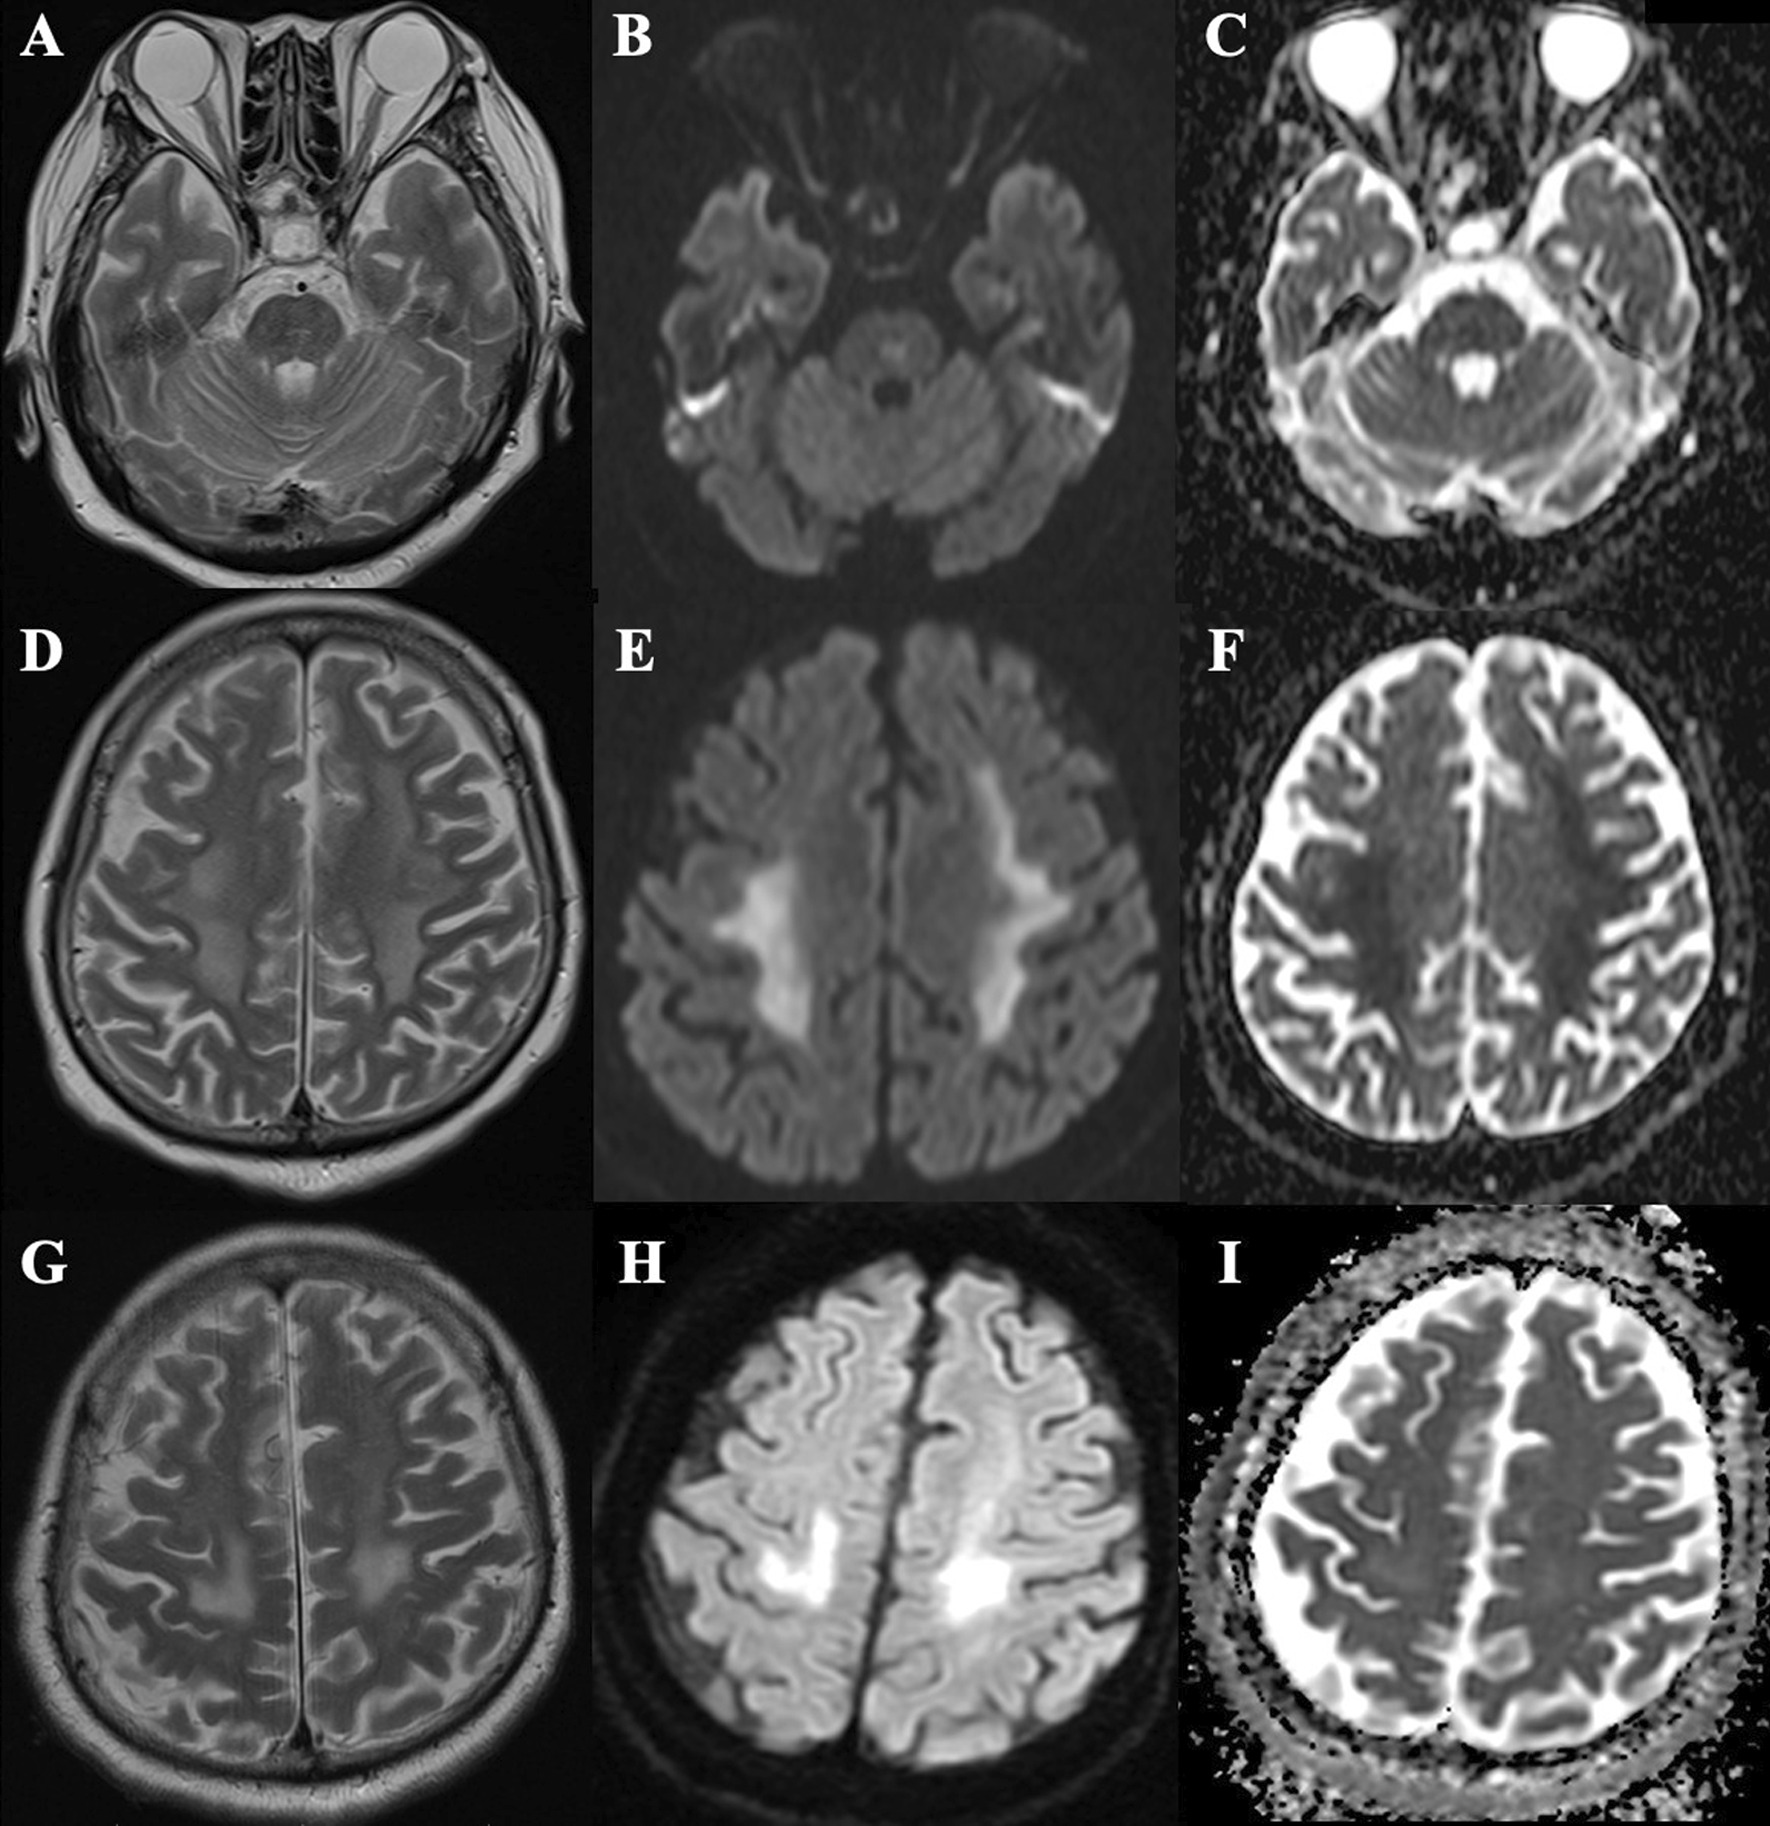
Brain magnetic resonance imaging (MRI) findings. A–C T2-weighted imaging (A) performed on day 25 reveals a lesion in the pons, with hyperintensity on diffusion-weighted images (B) and low apparent diffusion coefficient values (C). D–F T2-weighted imaging (D) performed on day 25 reveals symmetrical, bilateral periventricular deep brain white matter lesions, with hyperintensity on diffusion-weighted images (E) and low apparent diffusion coefficient values (F). G–I T2-weighted imaging (G) performed on day 70 reveals a reduction in the white matter lesions, with hyperintensity on diffusion-weighted images (H) without low apparent diffusion coefficient values (I)
